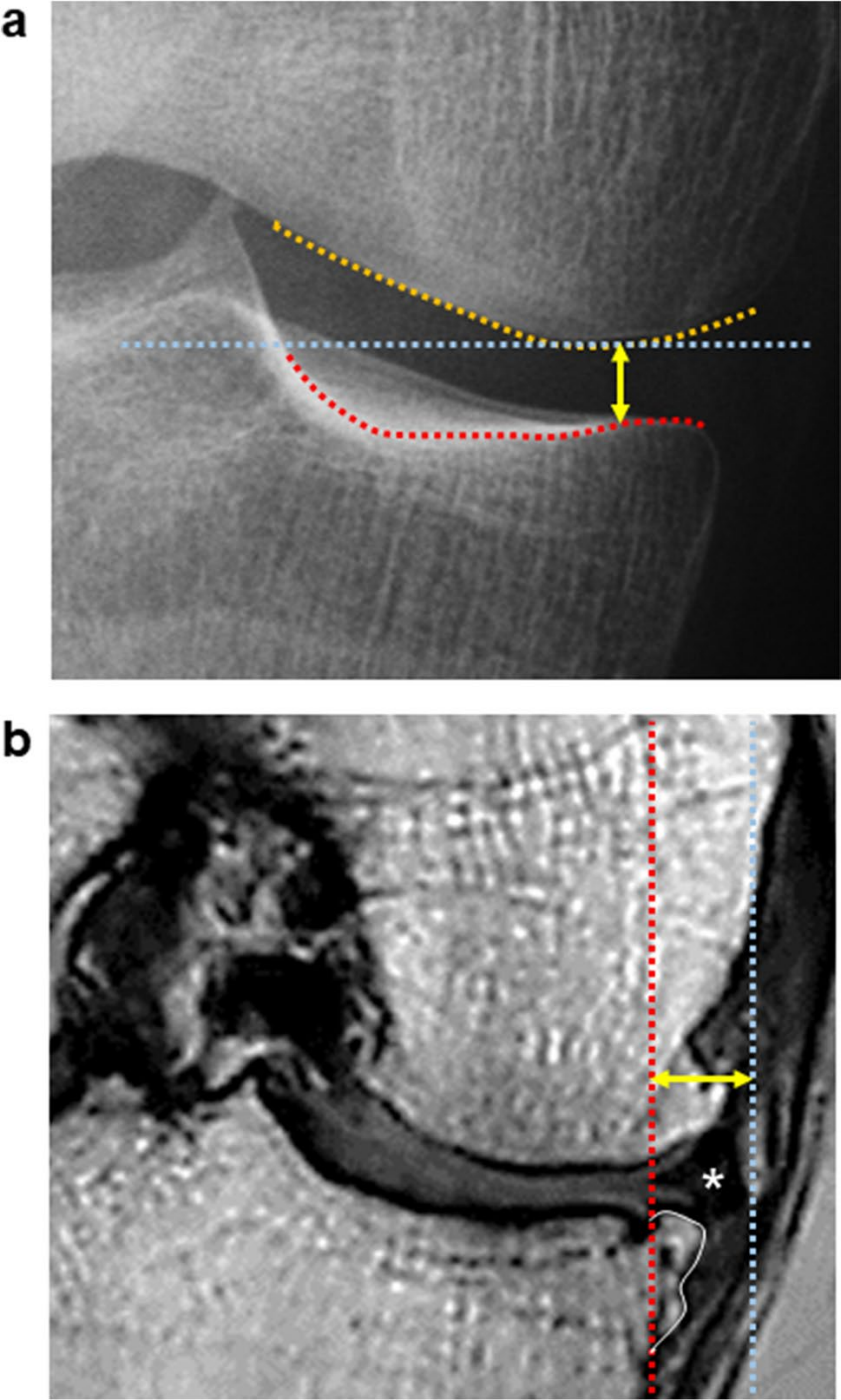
Measurement methods. a Measurement of the minimum joint space width by radiography. A horizontal straight line (blue line) was drawn at the lowest end of the medial femoral condyle (orange line). A bright radio-dense zone (red line), defined at the anterior edge of the medial tibia, was also drawn. The minimum distance (yellow arrow) between these two lines was defined as the minimum joint space width (mJSW). b Measurement of MME by MRI. Using a coronal plane MRI, the MME (yellow arrow) was defined as the distance between the perpendicular line (red line) from the medial margin of the tibia, excluding the osteophytes (orange line), and the perpendicular line (blue line) from the outer edge of the MM (star)
